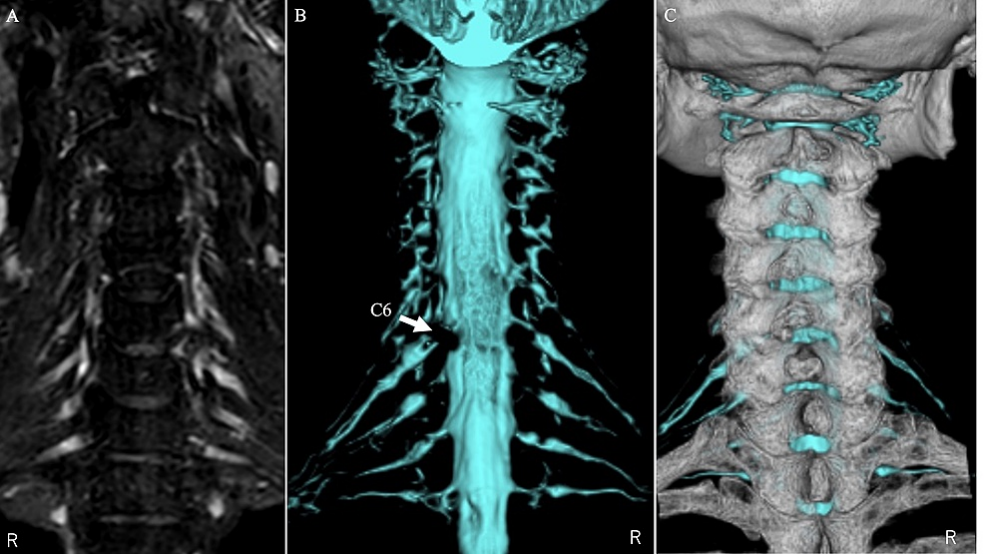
CT/MRI fusion images of case 1 The left image (A) shows the nerve root in the 3D STIR sequence. The middle image (B) is a 3D MRI image of the dural tube and the nerve root taken with a 3D STIR sequence on the left image. The right image (C) is a CT/MRI fusion image of the 3D CT image and the 3D MRI image.
